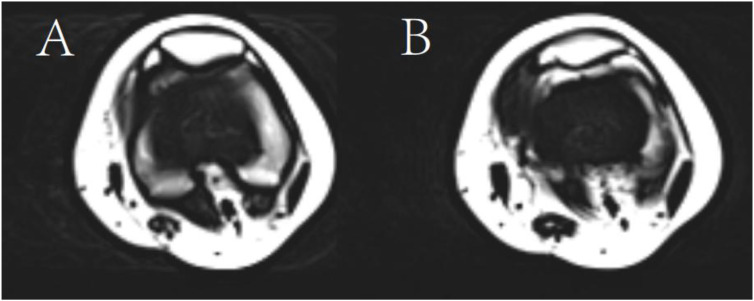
T2-weighted sequence. (A) Good Prognosis; (B) Poor Prognosis.
